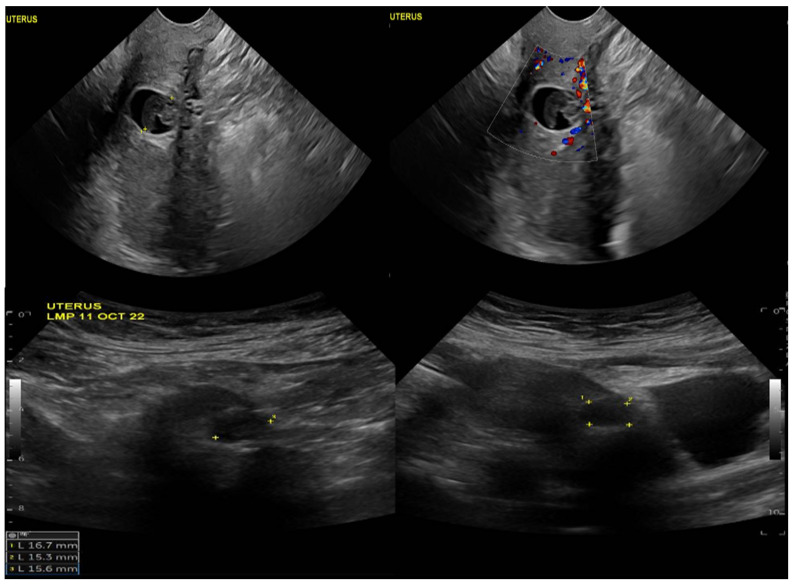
Ultrasound image of the uterus, demonstrating a well-defined ectopic gestational sac and showing a fetal pool with no definite pulsations in the anterior wall of the lower uterine segment at the assumed region of the previous scar.
